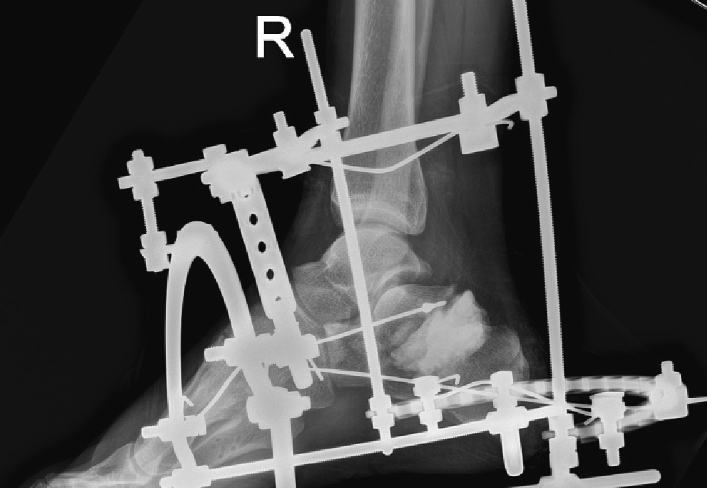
Post-operative lateral x-ray.
